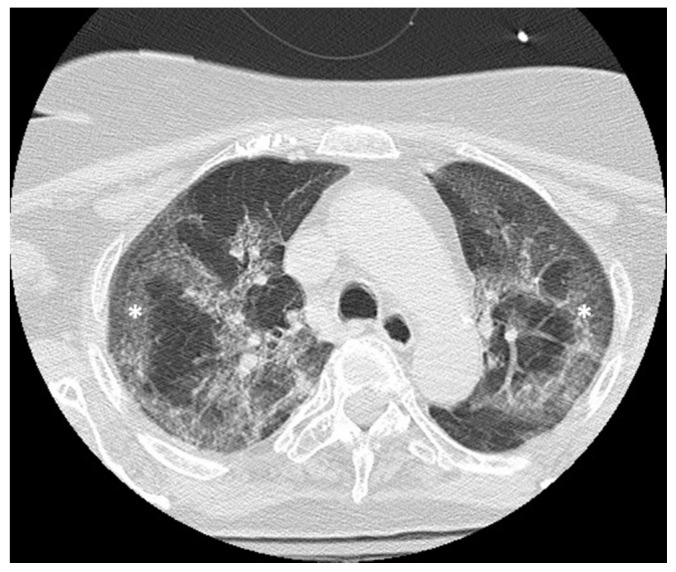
Axial CT image shows ground-glass opacity superimposed by interlobular and intralobular septal thickening (asterisk).
